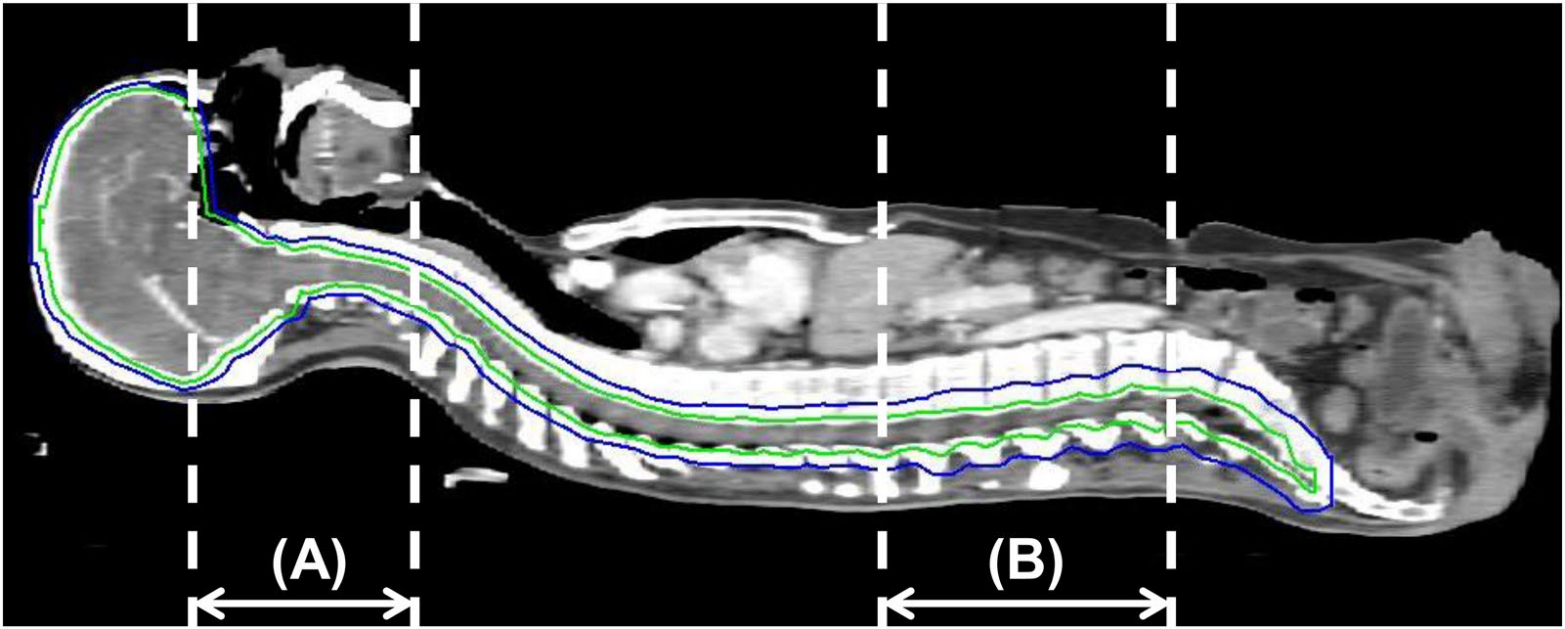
Target delineation for craniospinal irradiation (green line, clinical target volume [CTV]; blue line, planning target volume [PTV]) (A) Range of daily pre-treatment megavoltage computed tomography (MVCT). (B) Range of daily post-treatment MVCT.
